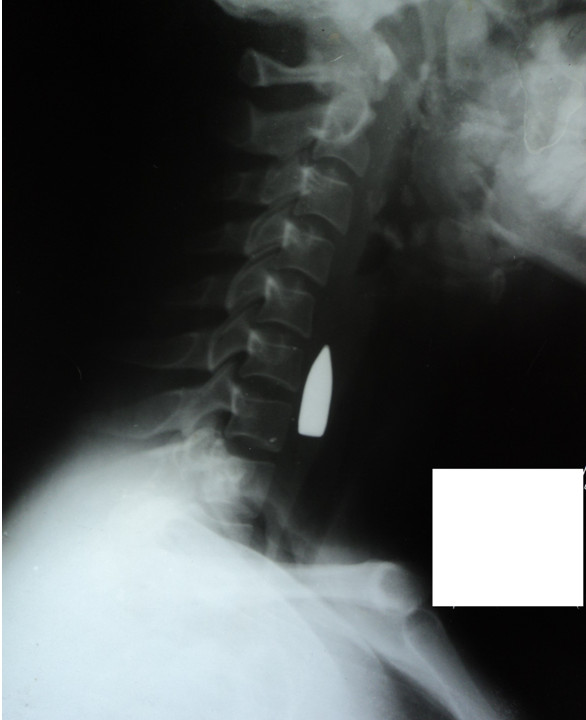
Lateral radiograph of the head and neck. This shows a bullet lodged in the neck, anterior to the bodies of cervical vertebrae 6 and 7.
